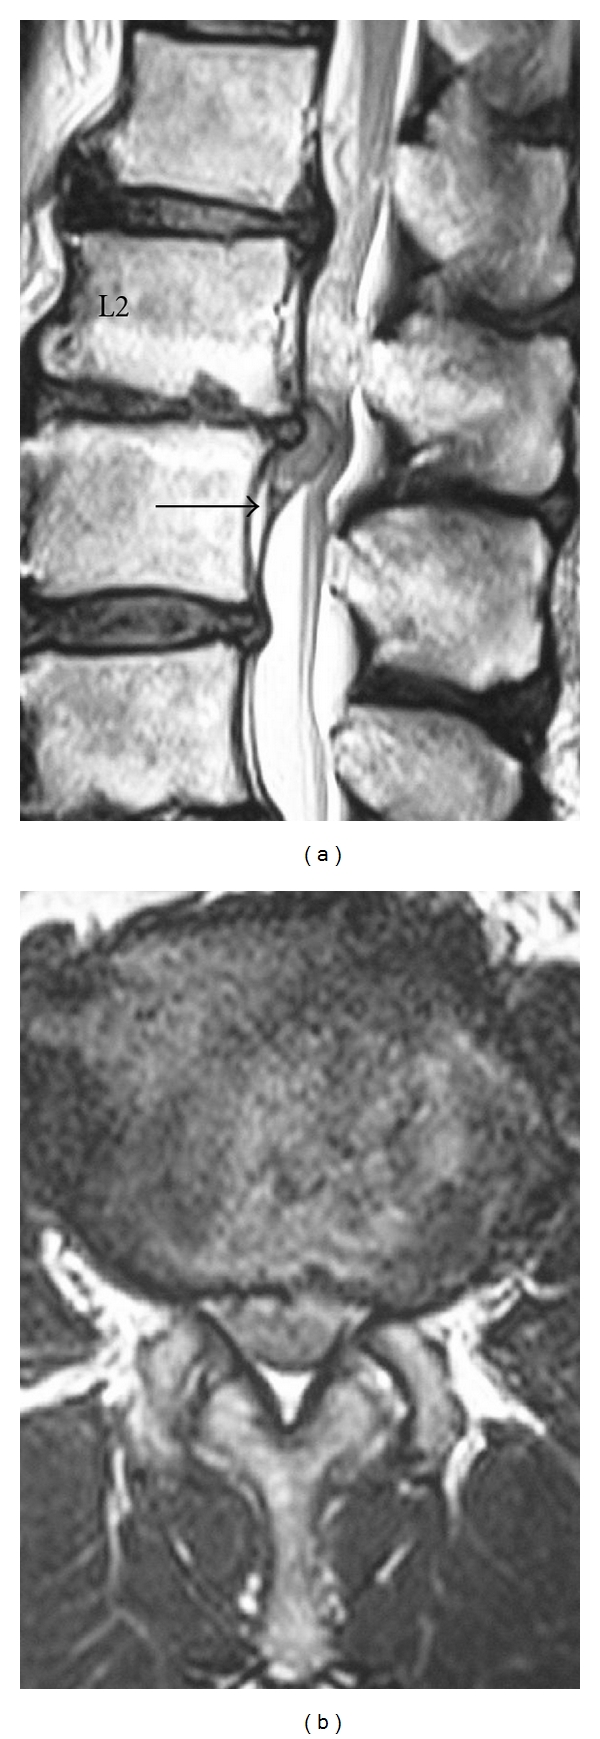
Preoperative MRI of the lumbar spine: (a) sagittal plane T2-weighted image. (b) Axial plane T2-weighted image. The ventral dural line was divided such that disc herniation existed between the two lines (black arrow) (a). The border of cauda equina and disc herniation was unclear (b).
